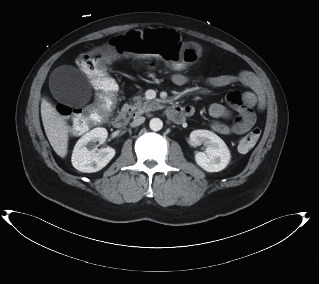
CT abdomen image showing multiple shotty mesenteric lymph nodes, which are nonspecific, possibly reactive related to the colonic process.
